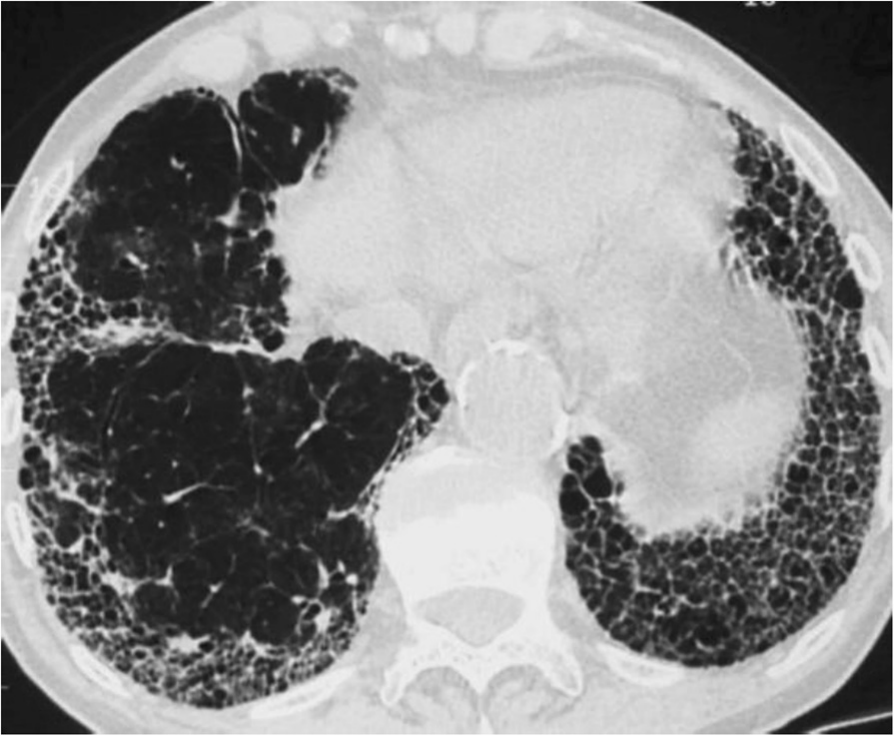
CT scan of thorax in a patient with IPF showing typical basal, sub-pleural, honeycomb shadowing and traction bronchiectasis.
